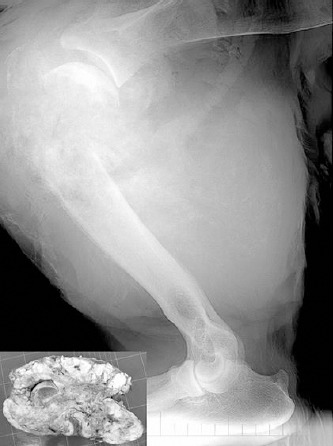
Mediolateral radiograph shows a destructive bone lesion with pathological fracture of the proximal third of the humerus. The tumor is densely sclerotic, containing a considerable amount of mineralized osteoid. Small picture in the inset shows gross appearance of the osteosarcoma, with destruction of the cortex and a soft tissue mass with osteogenic white areas.
